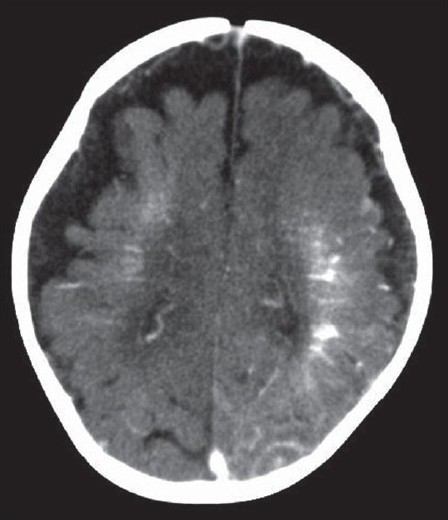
CT of head showing atrophy of brain and calcification of left parietal region.
